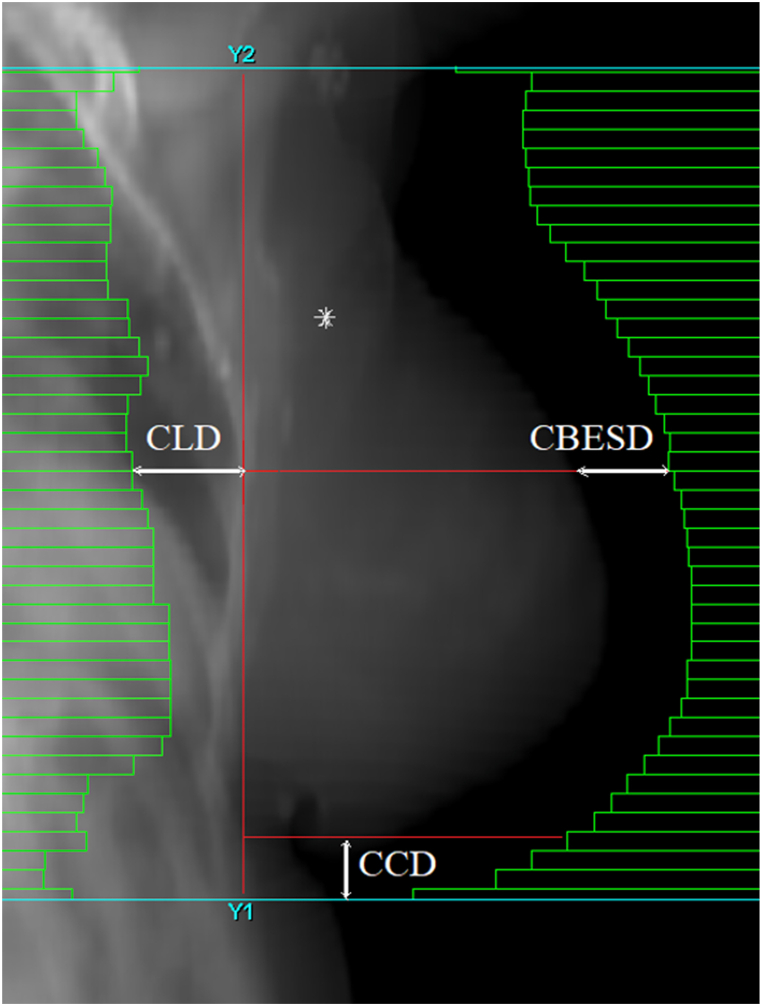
Tangential parameters evaluated in the Monaco 6.1.2 treatment planning system. The image is a digitally reconstructed radiograph showing the following tangential image parameters: CLD = central lung distance, CCD = central caudal distance, CBESD = central beam to edge skin distance.
